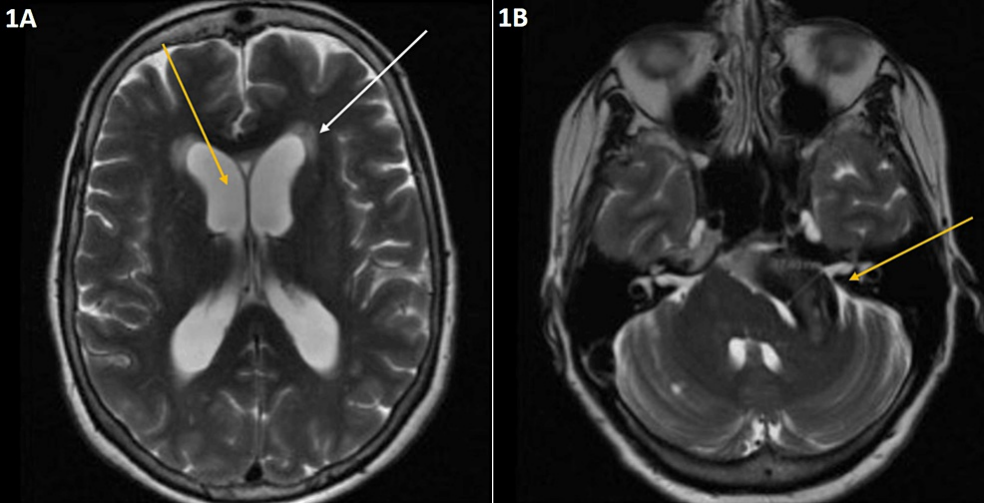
MRI T2 axial plane 1A. MRI T2 axial plane demonstrating dilated lateral ventricles (yellow arrow) with trans-ependymal transudation (white arrow). 1B. MRI T2 axial plane demonstrating a fusiform aneurysm compressing the pons (yellow arrow).
